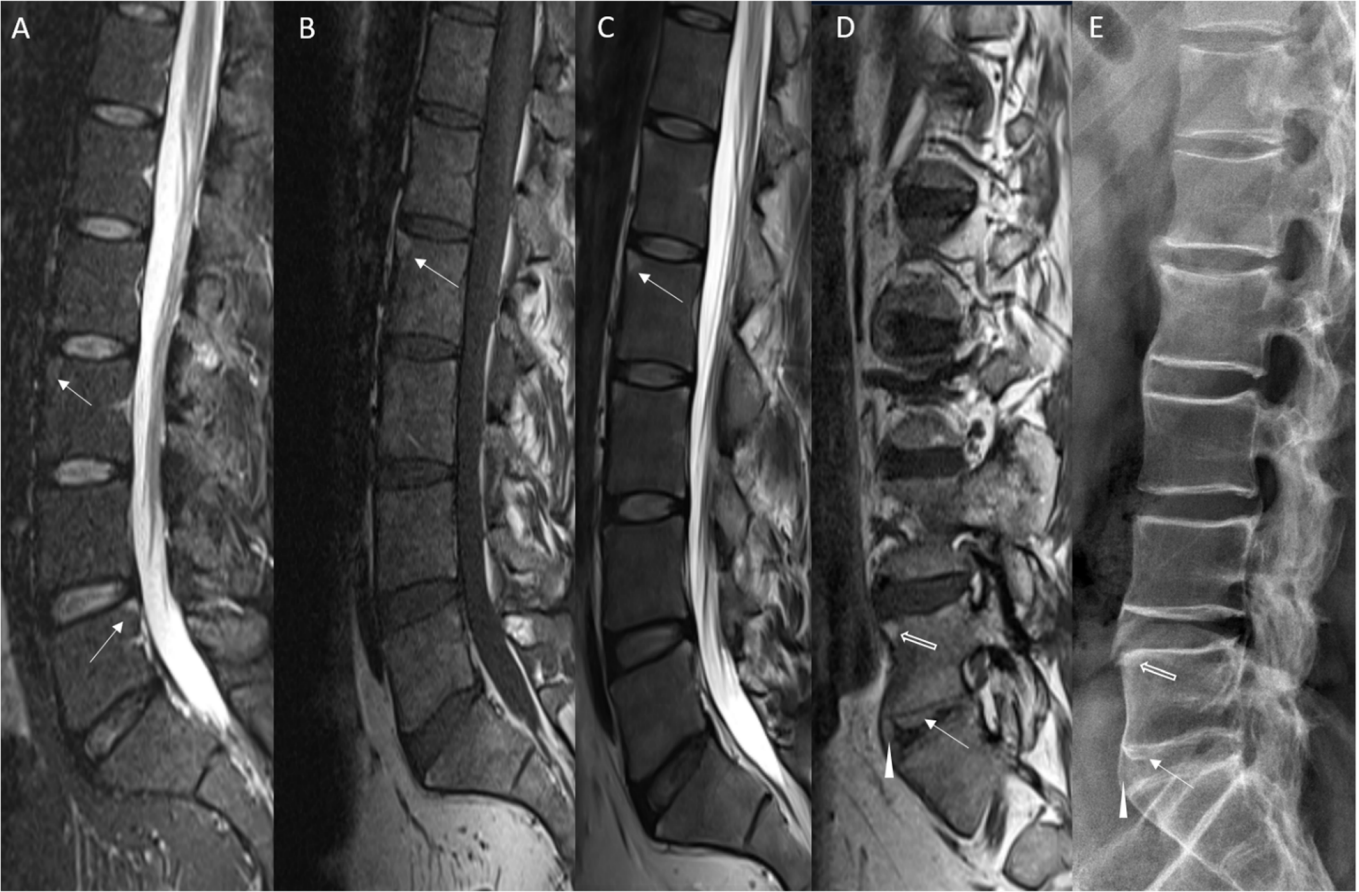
Multi-modality representation of typical imaging findings in ankylosing spondylitis (AS). Morphological MRI findings (a–d) and radiographical findings (e) are demonstrated. a–c Sagittal short tau inversion recovery (STIR, a), T1-weighted (T1w, b), and T2-weighted (T2w, c) images of the lumbar spine (T12–S2) of a 31-year-old male with r-axSpA. Typical disease-related changes are acute inflammation of vertebral corners (a) that are visible as multi-segmental focal signal hyperintensities of the anterior and posterior corners of vertebral endplates (white arrows in a). Signs of chronic inflammation, i.e., fatty infiltration, of the vertebral endplate corners are detected as signal hyperintensities in T1w and T2w images (white arrows in b and c). d, e Sagittal T1w image (obtained laterally at the height of the neuroforaminal openings) and lateral radiographic projection of the lumbar spine of a 46-year-old male with AS. Here, chronic inflammation at a vertebral endplate corner is visible as a focal signal hyperintensity (block arrow in d) or focal sclerosis (block arrow in e) of the upper anterior corner of the vertebral body of L5. New bone formations can be seen as bridging syndesmophytes (arrowheads) and as transdiskal ankylosis (white arrows)
